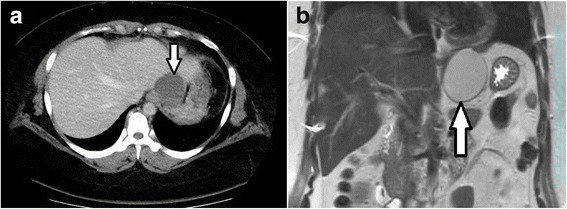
a Preoperative computed tomography showed the cystic tumor attached to the anterior wall of the esophagus and EGJ. b T2-weighted magnetic resonance imaging (*coronal view*) showed a high-intensity mass between the liver and stomach
